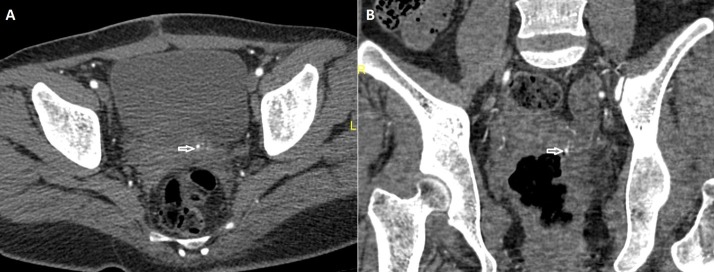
Coronal CT angiogram images of pelvis show contrast filling the pseudoaneurysm (arrowhead) within the uterus in relation to the left uterine artery.
